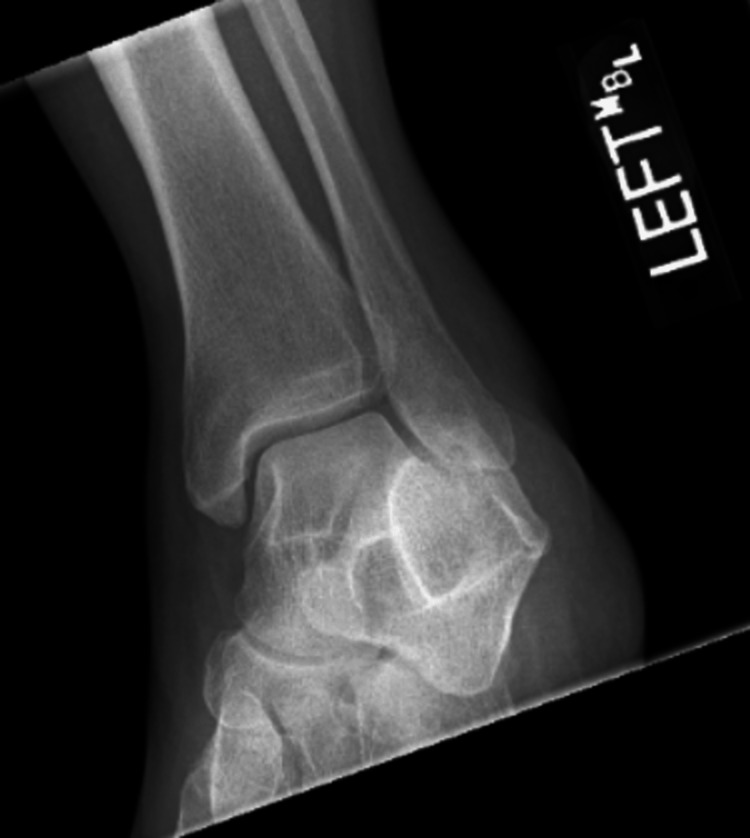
X-ray of left ankle showing soft tissue swelling and small ankle effusion
